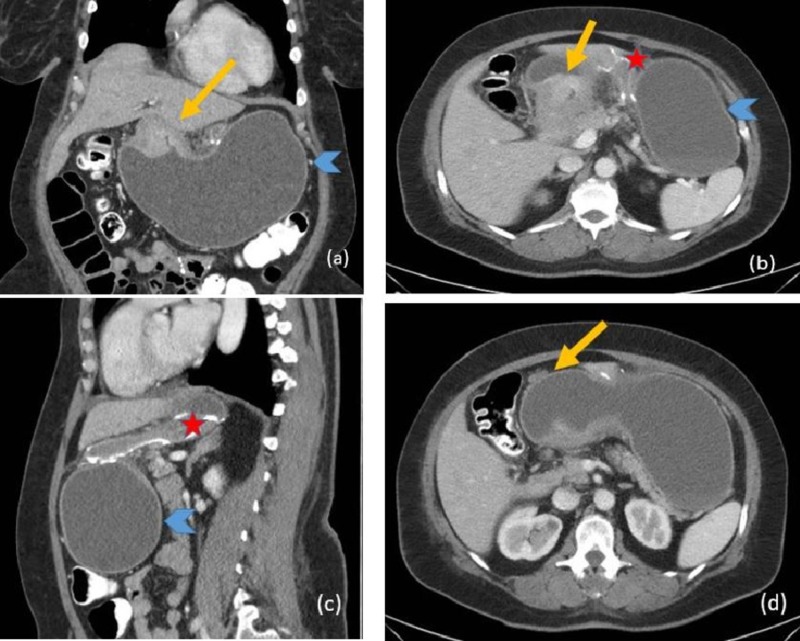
Abdominal computed tomography (CT) scan A-C show an obstructing, enhancing soft tissue mass at the pylorus measuring approximately 5.5 x 4.5 cm (yellow arrow). There is associated marked fluid-filled distention of the gastric remnant (blue arrowhead). Normal appearance of the Roux-en-Y gastric bypass (star). (D) Contrast-enhanced axial CT image at the level of the upper abdomen demonstrates a small soft tissue nodule anterior to the gastric antrum; indeterminate but suspicious for peritoneal metastatic disease.
